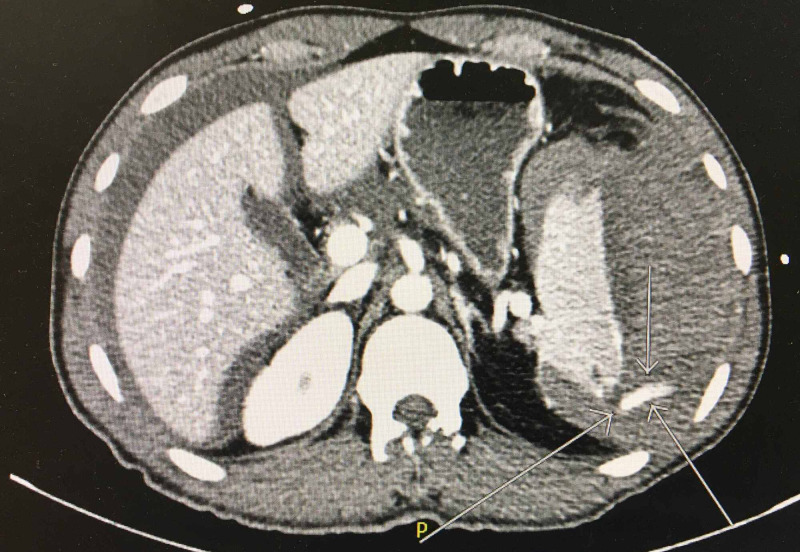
CT showed linear hyper-density extending from the posterior aspect of the spleen (arrows) compatible with active extravasation likely due to active bleeding.
